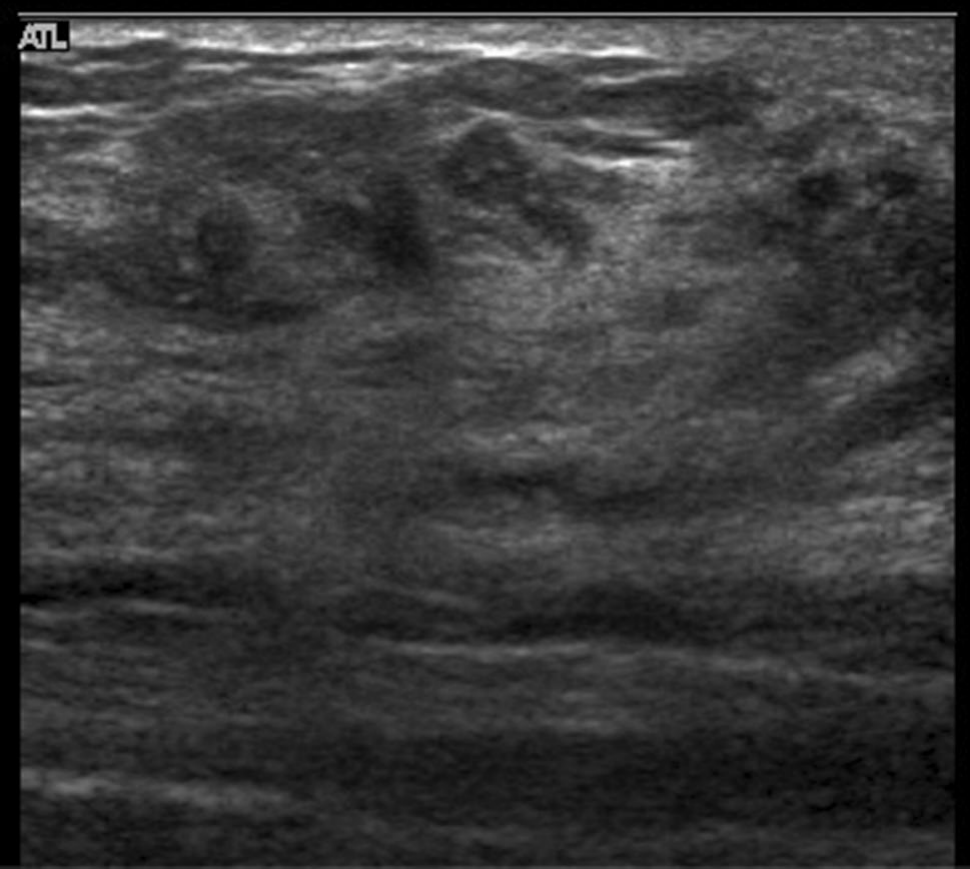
Case of ductal carcinoma in situ (DCIS). Ultrasound shows a patchy hypoechoic area in the mammary gland
